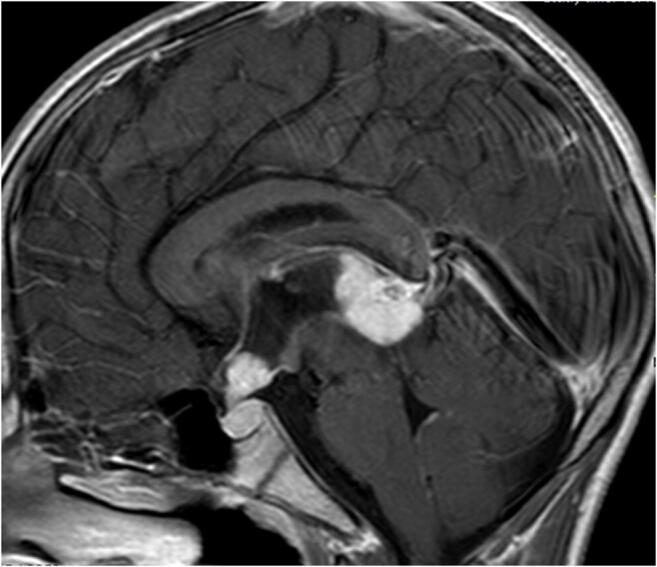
Bifocal (suprasellar/pineal) germ cell tumor (BGCT): sagittal T1-contrast-enhanced MRI of a 13-year-old boy presenting with obstructive hydrocephalus and diabetes insipidus. MRI shows a “bifocal–pineal and suprasellar” tumor. A shunt was placed. CSF and blood markers (AFP + BHCG) were negative. Patient was treated for a presumed germinoma and is tumor-free for more than 5 years. One year after shunt insertion, he underwent an endoscopic third ventriculostomy (ETV) due to a shunt malfunction
